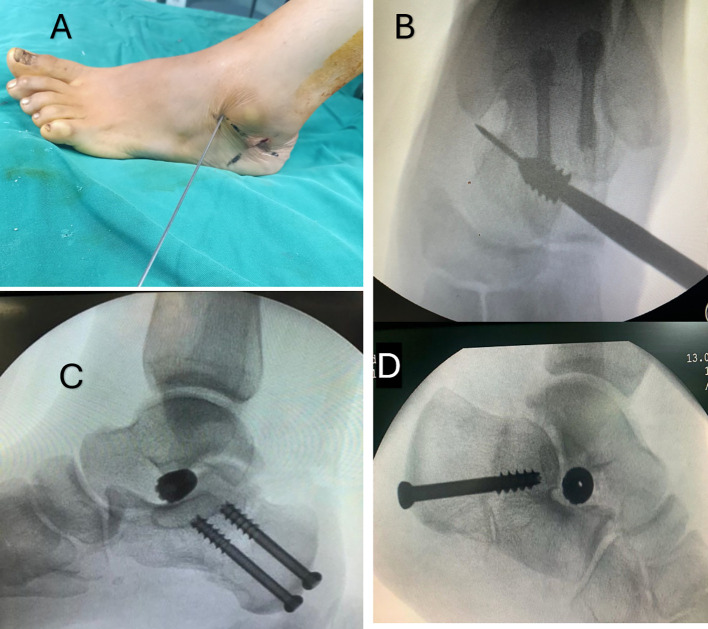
A: Intraoperative photograph of insertion of STA guidewire into tarsal canal. B: Intraoperative X-ray of insertion of STA screw over the guidewire. C,D: Final intraoperative X-ray after combination MDCO + STA in 2 different cases.
